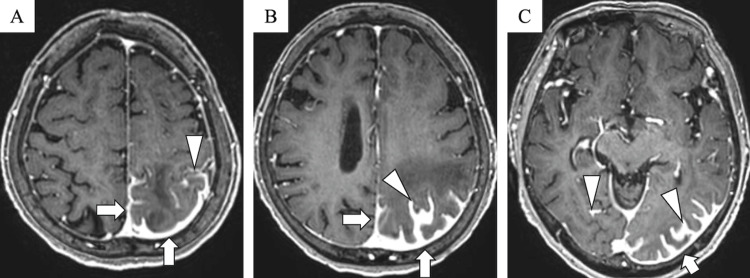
Contrast-enhanced T1-weighted imaging. Contrast-enhanced T1-weighted imaging shows leptomeningeal enhancement extending from the parietal to the occipital lobe (A–C, arrowhead) and enhancement of the adjacent dura mater (A–C, arrow). No parenchymal enhancement is observed.
